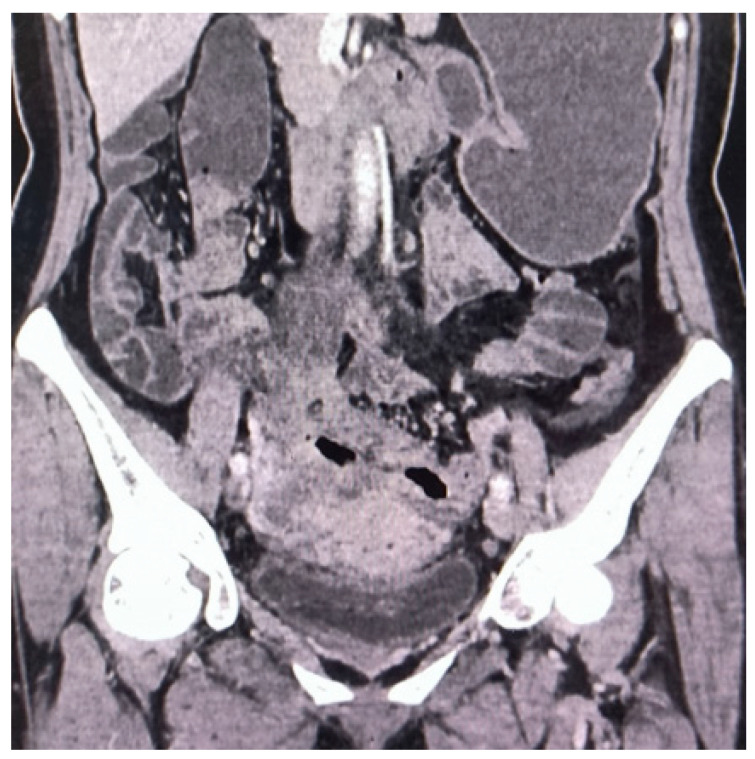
Contrast-enhanced computed tomography (CT) revealed roughening and thickening of the sigmoid colon and upper rectum walls, with luminal narrowing and uneven enhancement. In conjunction with laboratory examination (RBC 2.95 × 10^12^/L, Hb 80 g/L, WBC 12.3 × 10^9^/L, CRP 53.44 mg/L, CEA 1.73 ng/mL, AFP3.64 ng/mL, CA125 19.2 U/mL, CA19-9 8.83 U/mL), CT indicated possible inflammatory lesions with pelvic lymph node enlargement.
